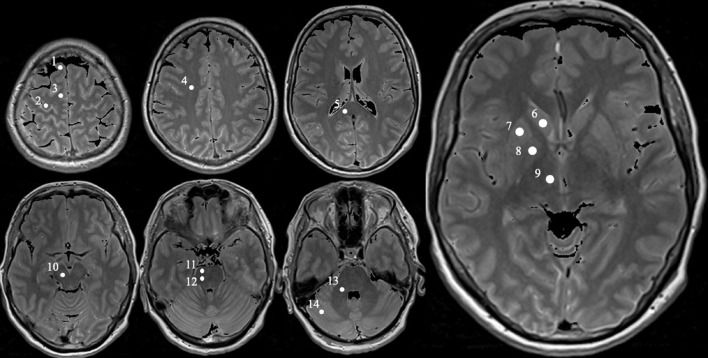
Exemplary MRI with definition of the specific measured regions obtained from a PD patient (female, 62). Positions of each selected ROI in the right brain hemisphere marked as white circle on proton density weighted MR images obtained from a 62-year-old female Parkinson’s disease patient. The circles are numbered 1 to 14, representing the following ROIs: prefrontal subcortical area (1), subcortical primary motor area (2), supplementary motor area (3), centrum semiovale (4), splenium corporis callosi (5), nucleus caudatus (6), putamen (7), globus pallidus (8), thalamus (9), mesencephalon (containing substantia nigra and ventral tegmental area, 10), upper and lower pons (11 and 12, respectively), cerebellar white matter (13), and cerebellar posterior lobe (14).
